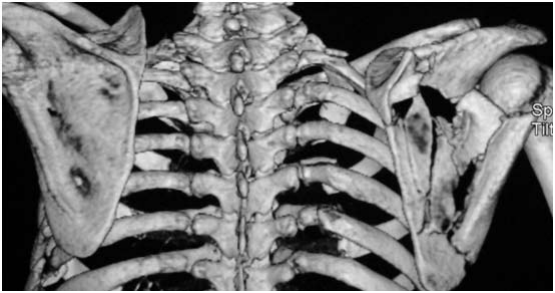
Fig. 3: CT scan axial image revealing fracture of multiple spinous processes with the fractured fragments drawn towards the side of the scapulothoracic dissociation demonstrating the avulsion fracture and direction of force. F3:
